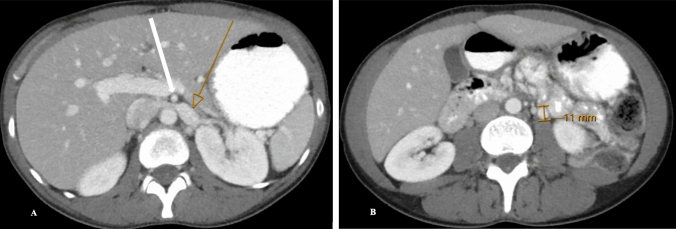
Aortomesenteric left renal vein compression in a patient with pelvic venous disorder. A 35-year-old multiparous female presenting with several months of abdominal pain and nausea. Axial contrast-enhanced computed tomography shows prestenotic dilatation of the left renal vein (orange arrow, Fig. 4A) with focal aortomesenteric narrowing between the superior mesenteric artery (white arrow, A) and aorta. The same imaging study shows a dilated left ovarian vein measuring 11 mm (B)
